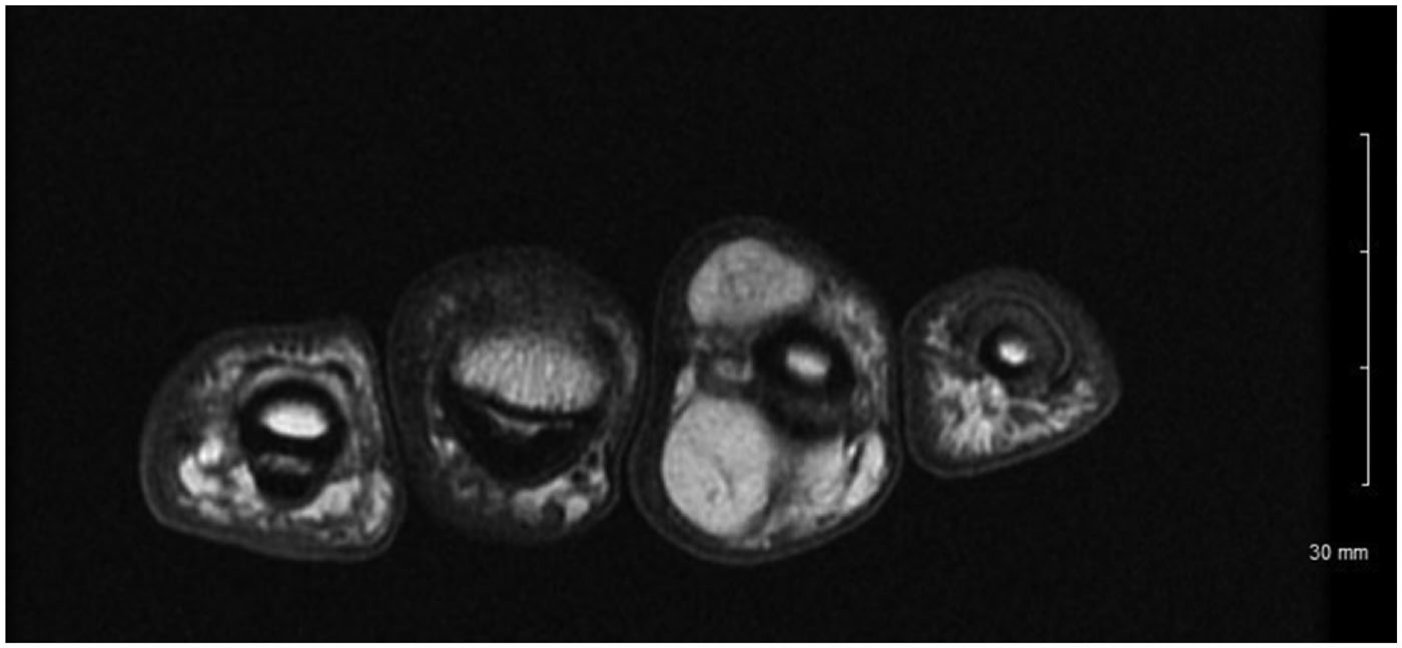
Axial MRI T1 weighted image with Fast Spin Echo (FSE) showing hyperintense lesion on both the volar and dorsal side of ring finger.
